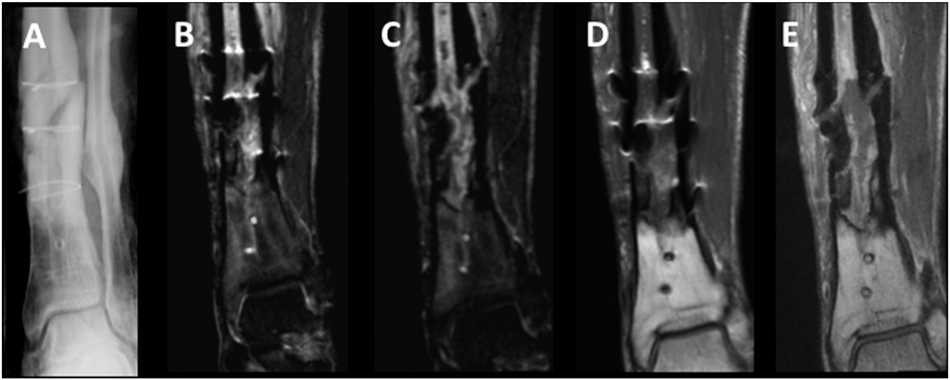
Infection. Posttraumatic pseudarthrosis at the left distal tibia with infection and intramedullary abscess formation in a 27 year old male patient with remaining cerclage fixation. (A) a.p. radiograph; (B) conventional coronal STIR image; (C) VAT&SEMAC coronal STIR image; (D) conventional coronal contrast enhanced T1-w image; (E) VAT&SEMAC contrast enhanced coronal T1-w image.
